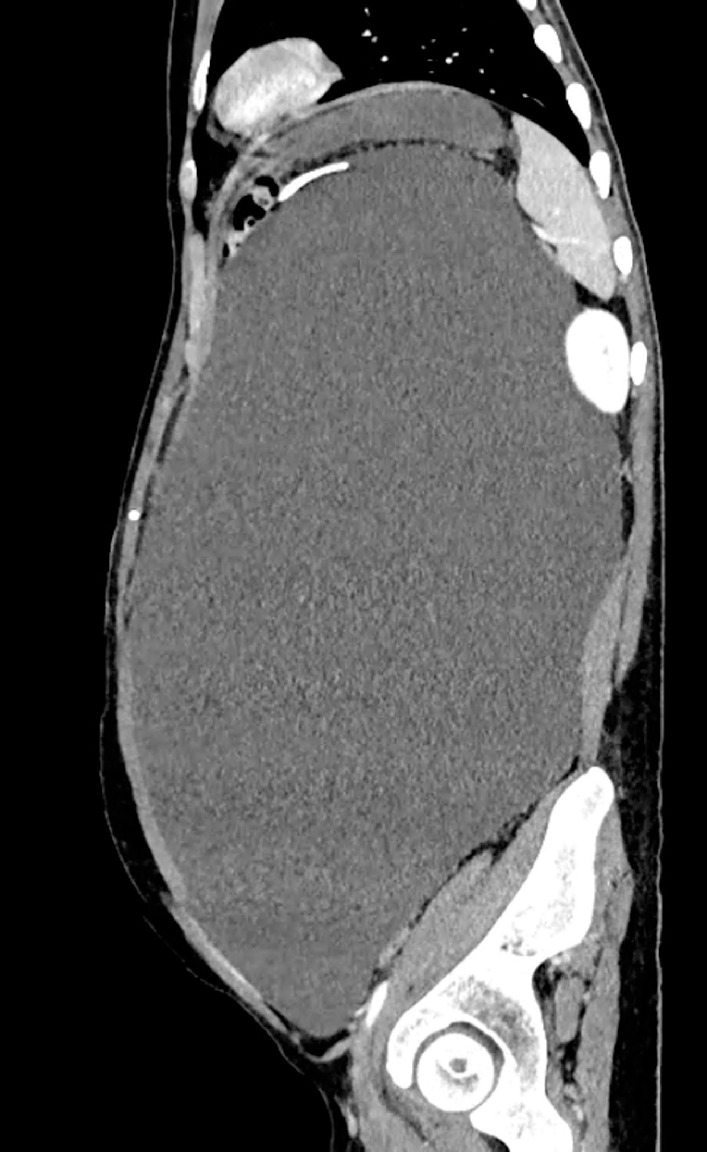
Computed tomography (CT) scan showing a large, encysted collection (16 × 25.6 × 41 cm) occupying the whole abdomen, displacing the bowel loops peripherally, with no free fluid and a left ventriculoperitoneal shunt entering the collection.
